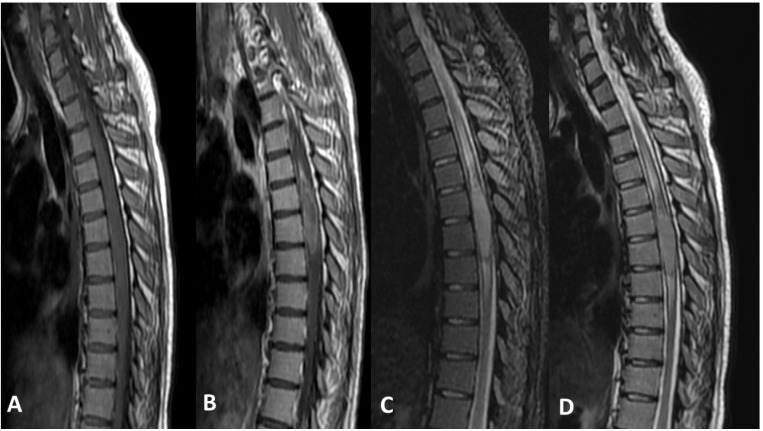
(A) Sagittal T1-weighted pre-IV contrast sequence of the dorsal spine. (B) Sagittal T1-weighted post IV contrast sequence of the dorsal spine. (C) Sagittal STIR sequence of the dorsal spine. (D) SagittalT2-weighted sequence of the dorsal spine.
